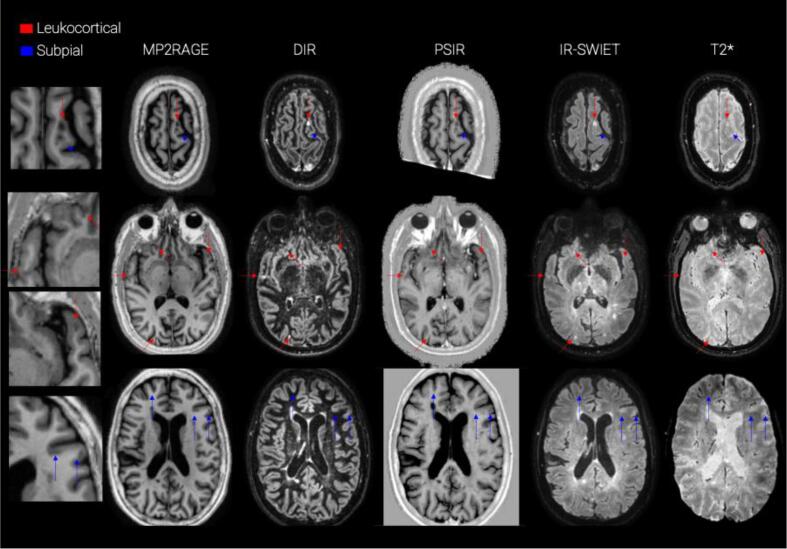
examples of CL seen in different MRI contrasts at 3 T. From left to right: MP2RAGE, DIR, PSIR, IR-SWIET, T2*. Red arrows point to leukocortical lesions and blue arrows to subpial lesions. (For interpretation of the references to colour in this figure legend, the reader is referred to the web version of this article.)
